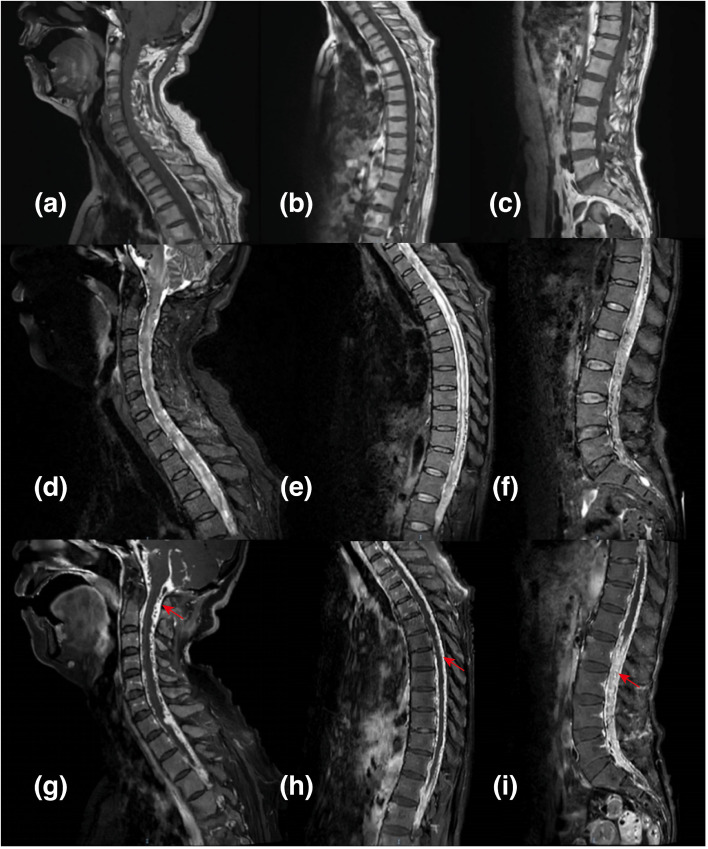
Spinal MRI before biopsy. Strip-like shadows with long T1 (a–c) and T2 (d–f) signals are visible throughout the spinal cord, without enhancement (g–i). The dura mater surrounding the spinal cord shows significant uneven thickening, appearing as long T1 (a–c) and T2 (d–f) signal shadows, with uneven enhancement [(g–i), red arrows].
